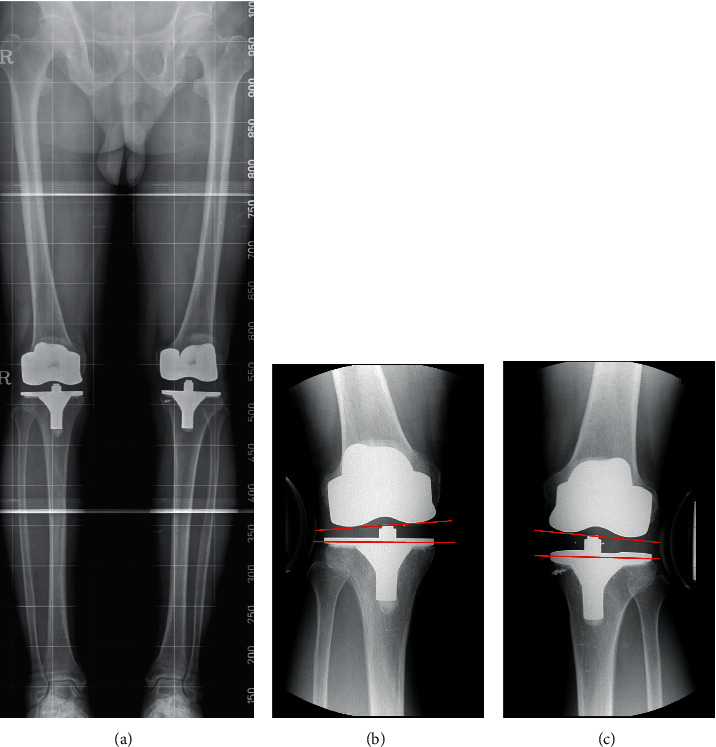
(a) Postoperative teleroentgenography. (b) Valgus stress radiographs of right knee extension; (c) Valgus stress radiographs of left knee extension.
